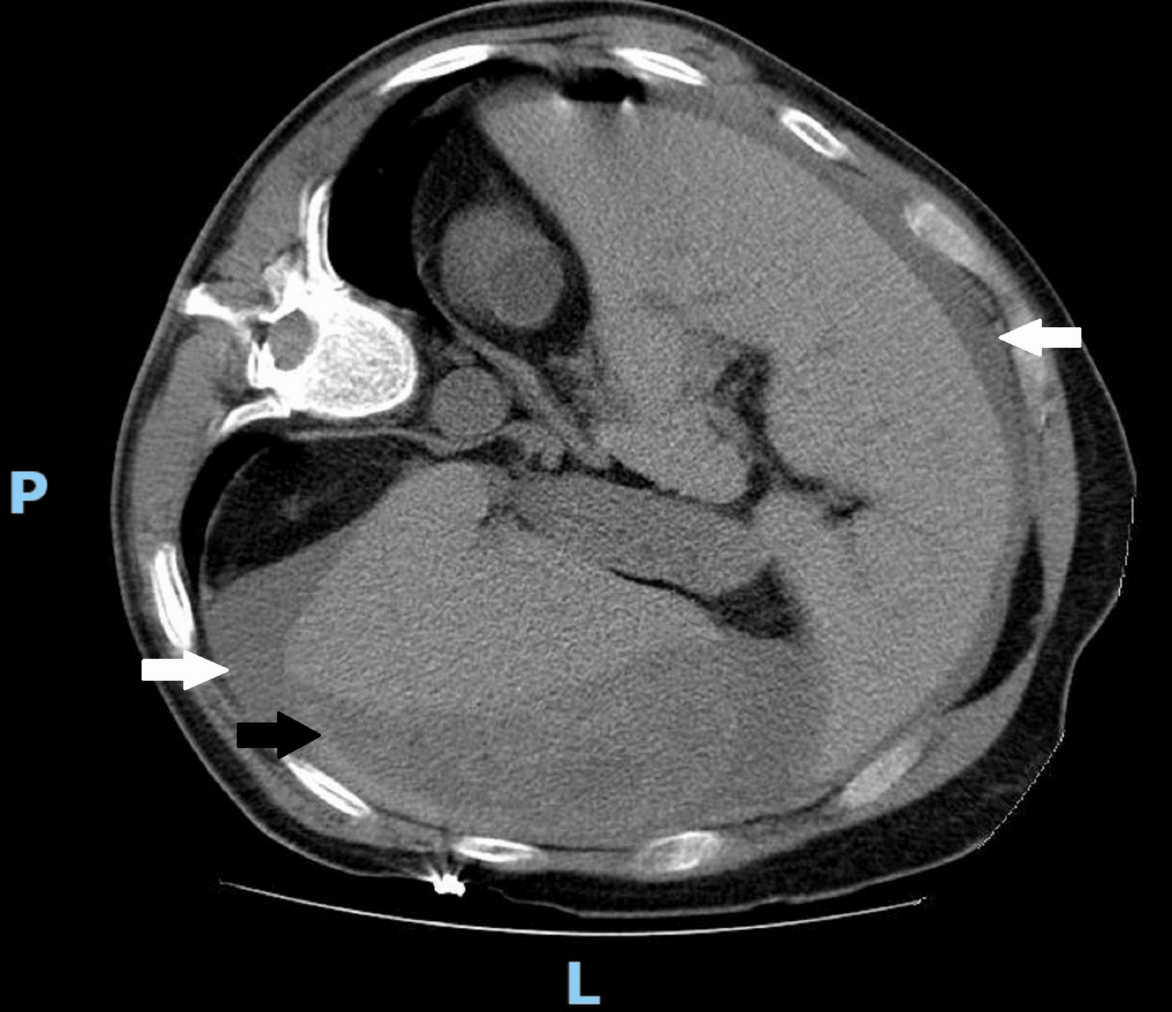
Abdominal computed tomography showing large hemoperitoneum (white arrows) and splenic subcapsular hematoma (black arrow) L: left; P: posterior
